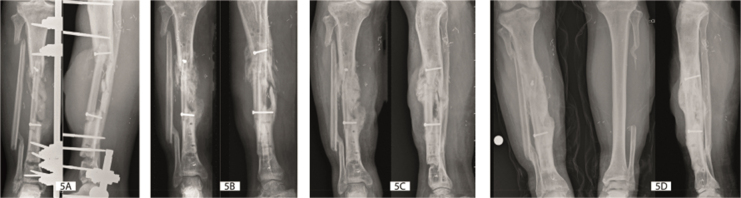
A. Tibial reconstruction using a vascularized fibular flap following a defected open fracture. Early postoperative images demonstrate new bone formation around the fibular flap, indicating a preserved periosteum at the reconstruction site. B. X-rays taken during the 5^th^ postoperative month reveal that the flap is united with the host bone, with gradual maturation of the peripheral bone wrapping, especially on the posterior and medial sides of the defect. C. X-rays taken during the 7^th^ postoperative month reveal a stress fracture of the flap, which facilitates new bone production at the flap’s anterior side. D. X-rays taken during the 35^th^ postoperative month show that this dual bone production generated massive hypertrophy, almost doubling the size of the reconstructed bone.
